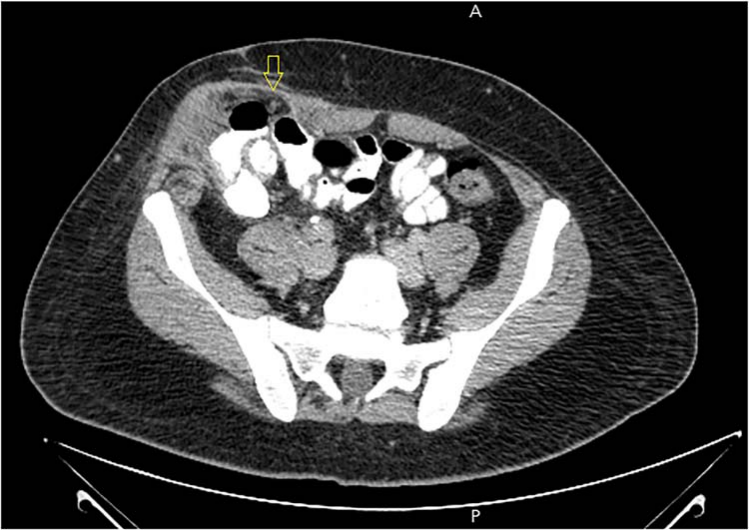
Abdomen CT scan with IV contrast demonstrated a large m peritoneal defect containing cecum and terminal ileum with an oval fatty density 1.5*2 cm represent an inflamed epiploic appendages (arrow) that have a hyper-attenuating rim and “central dot sign” with inflammatory changes in the adjacent fat tissue.
